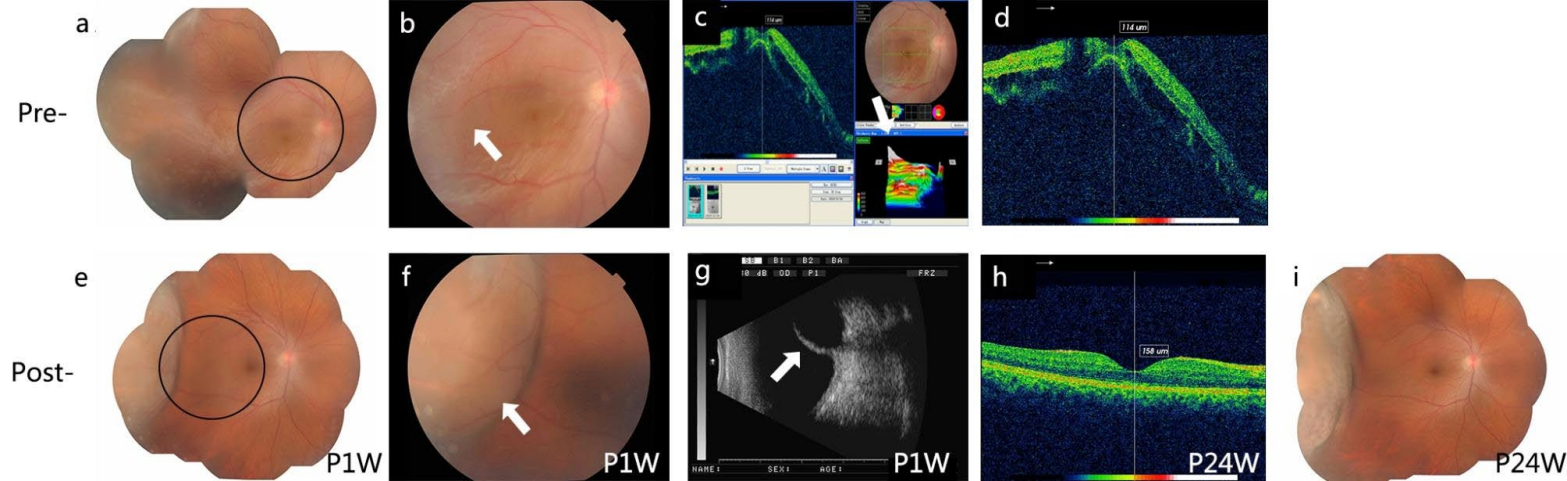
FCB scleral buckling efficacy on patient 2 (a) Fundus image showing retinal detachment pre-surgery. (b) The amplified circle area from A shows the retinal tear (white arrow). (c) Pre-operative B-ultrasound scan showing retinal detachment (white arrow). d–e. OCT shows an affected and detached macula. f. Fundus image of FCB scleral buckling at 1-week post-surgery (P1W). g. Fundus image showing clear and distinct photocoagulation spots (white arrow). h. Post-operative B-ultrasound scan results showing the FCB’s apparent pressure on the sclera (white arrow). i. OCT was showing the reattached macular. j. Fundus image at 24 weeks post-operation (P24W) showing the well-reattached retina
